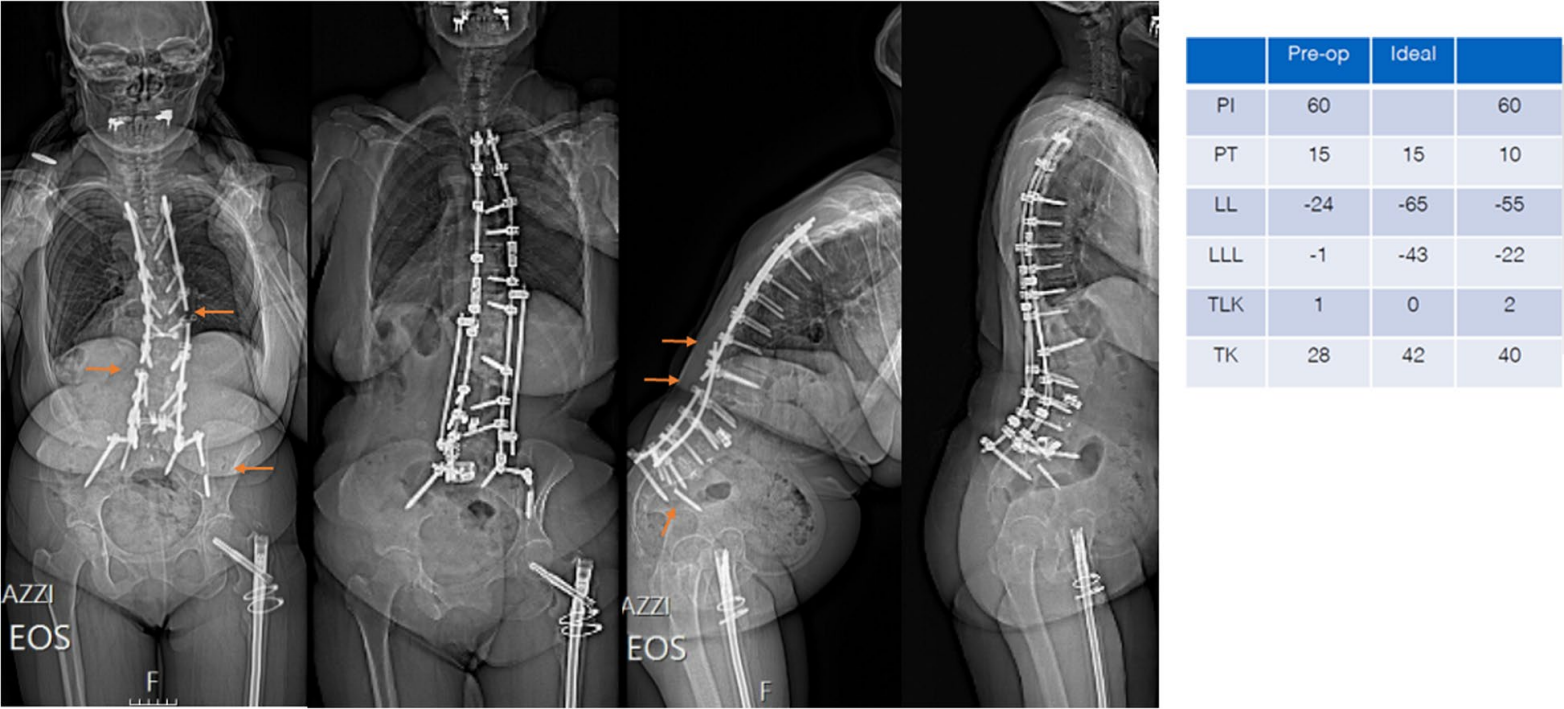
Pre- and postoperative x-ray following revision surgery in January 2021. The preoperative imaging showed multiple rod breakages and the breakage of the left iliac screw (arrows). A T4-pelvis revision with implant renewal, posterior release, hyperlordotic ALIF and 4-rod-construct was performed. After revision surgery LL and TK were restored. However, a deficit in LLL was observed
